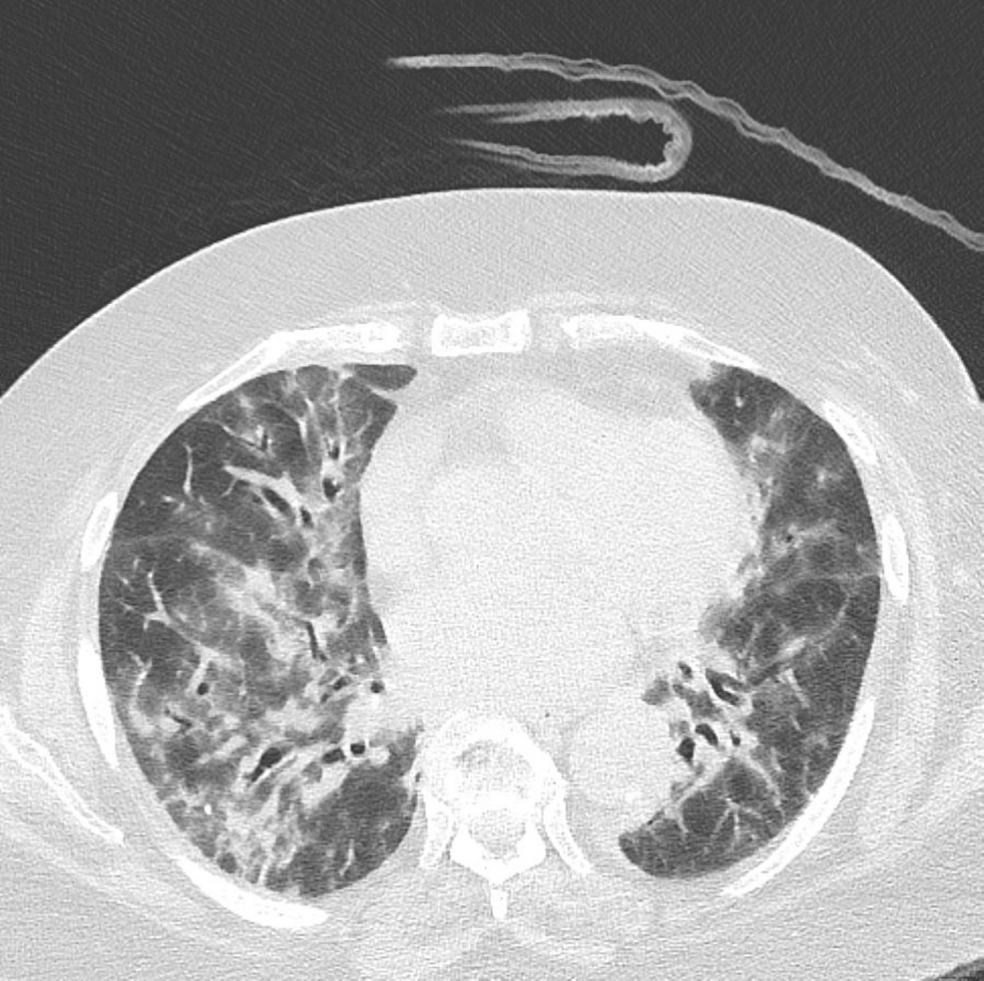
CT thorax showing bilateral patchy ground glass opacities associated with interlobular septal thickening, peri bronchial consolidations, and traction bronchiectasis (middle and lower lobes)
